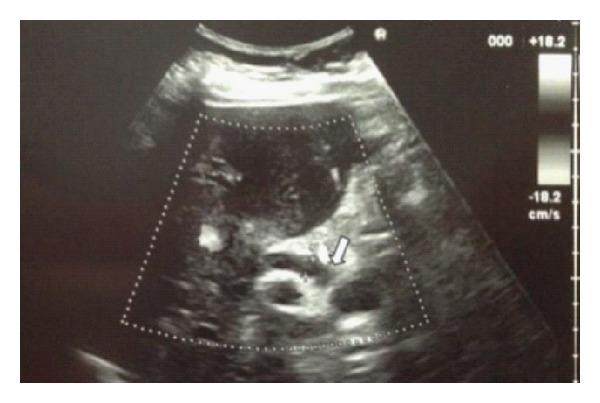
Abdominal ultrasound showing compression of the common hepatic duct which was dilated (8 mm) in its extrahepatic tract above the level of the obstruction (white arrow) and not sonographically observable under the level of obstruction.
